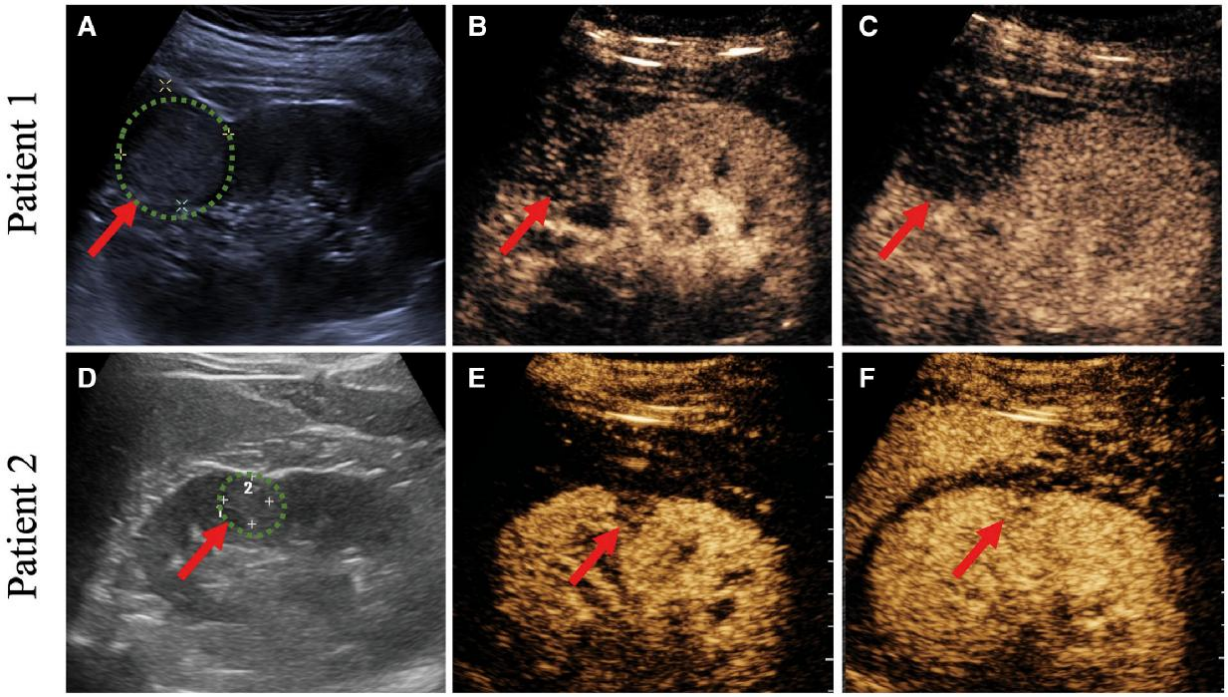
Contrast-enhanced ultrasound of two patients. (*A*) Greyscale ultrasound showed a circular, slightly hyperechoic mass measuring 32 × 35 mm in the mid-to-upper pole of the right kidney. (*B–C*) Contrast-enhanced ultrasound showed hypoperfusion within the mass in the cortical (*B*) and medulla phase (*C*). (*D*) Greyscale ultrasound showed a hypoechoic lesion in the upper pole of the kidney, ∼14 × 15 mm in size. (*E–F*) Contrast-enhanced ultrasound showed hypoperfusion within the lesion in the cortical (*E*) and medulla phase (*F*).
